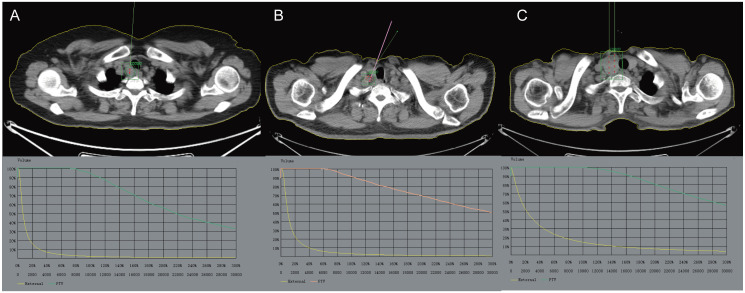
The preoperative CT scan images are imported into the TPS system for design, with the aim of avoiding important tissues and organs (such as large blood vessels, heart, spinal cord, etc.) as much as possible. (A) Enlarged right cervical lymph nodes with increased metabolism, indicating possible lymph node metastases. (B) Multiple lymph nodes in the right neck are enlarged and some of them have increased metabolism. (C) Occupation of the right lobe of the thyroid gland with increased metabolism is considered a local recurrence of thyroid cancer after surgery. The GTV and PTV are outlined. TPS, Treatment planning system; GTV, Gross Tumor Volume; PTV, Planning Target Volume.
